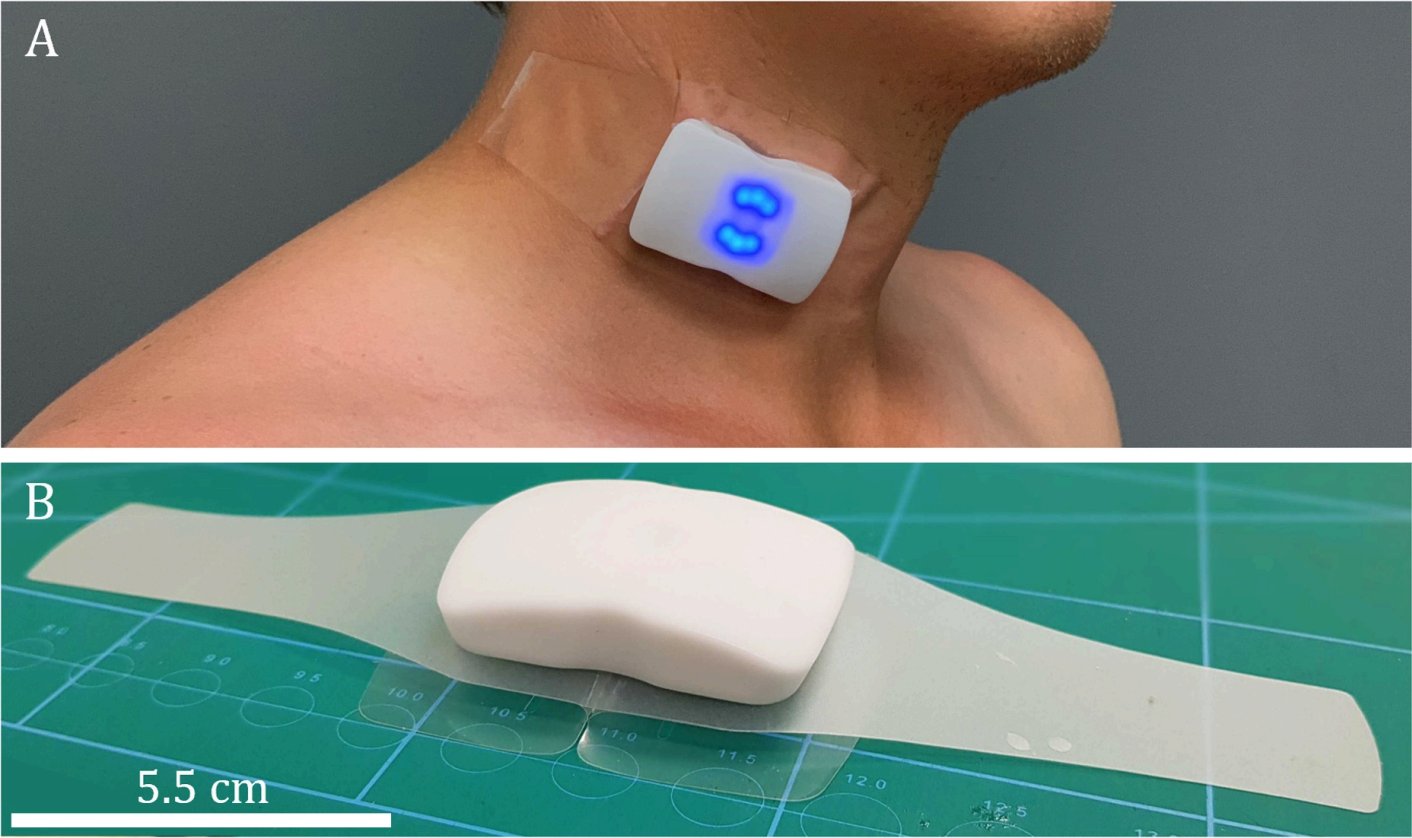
A and B: The wireless Doppler ultrasound patch.
